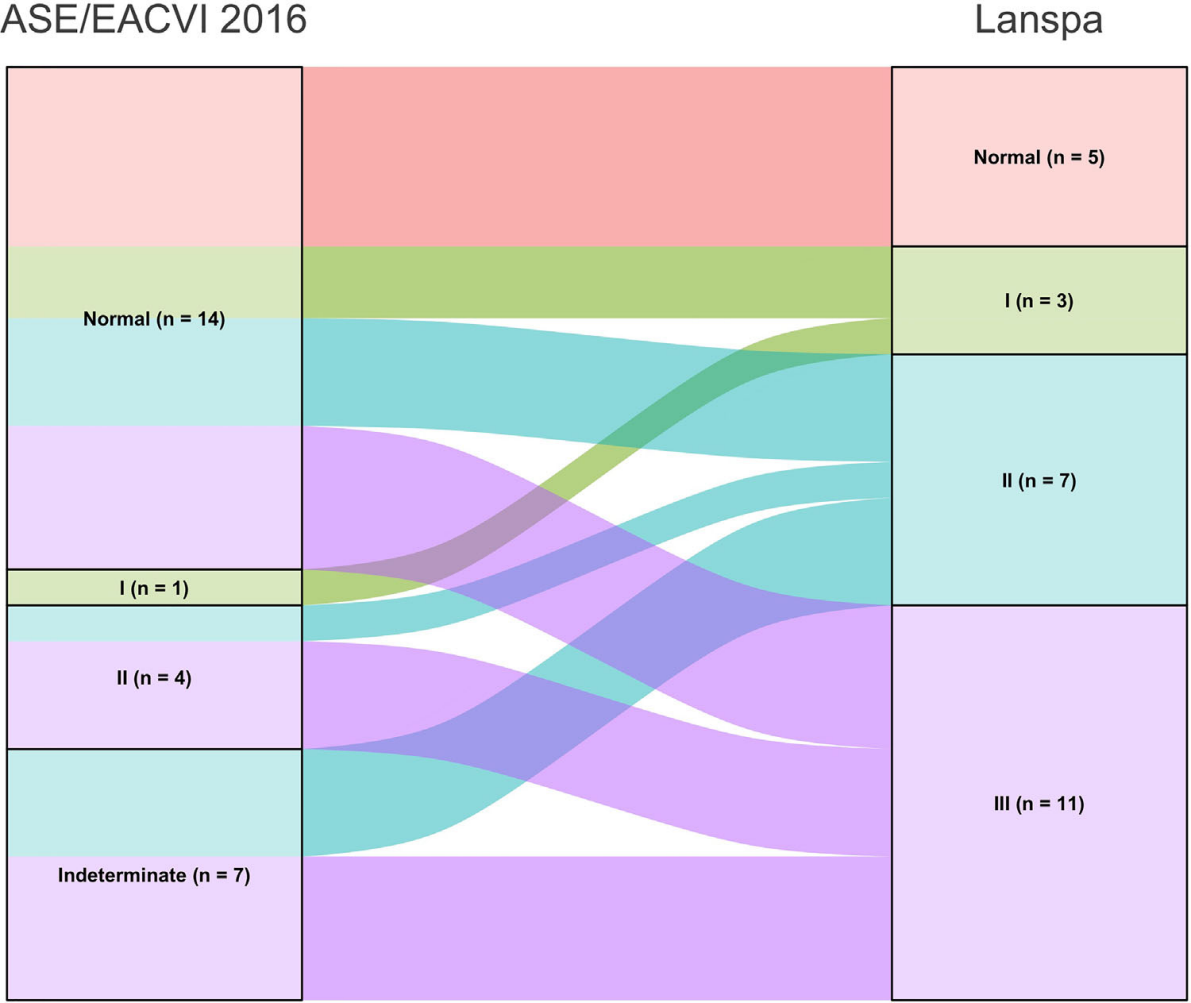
Alluvial Plot describing differences in diagnosis and grading of left ventricular diastolic dysfunction according to the two definitions. ASE, American Society of Echocardiography; EACVI, European Association of Cardiovascular Imaging.
